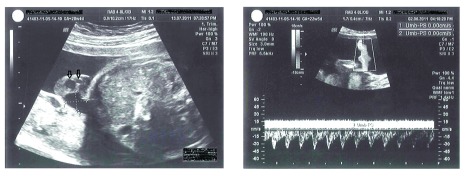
Traditional and Doppler ultrasound assessment showed only one umbilical artery (arrows).
